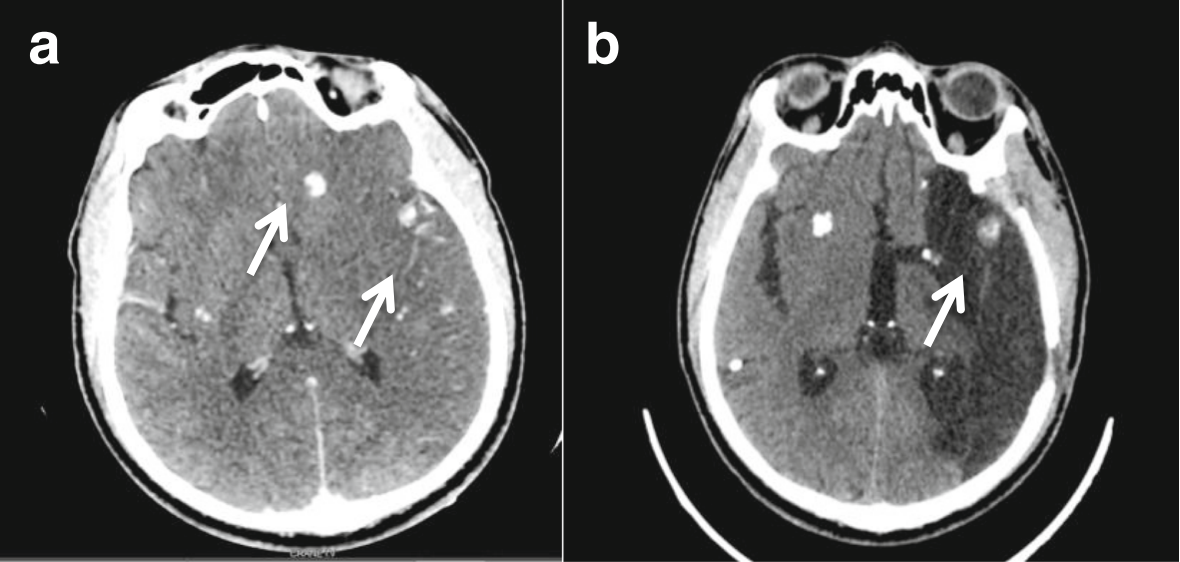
Brain CT of patient 3 before and after treatment. a Contrast-enhanced CT, calcified parenchymatous and subarachnoid cysticercus cysts within ischemic territory; b Calcified cysts within ischemic territory after craniectomy
